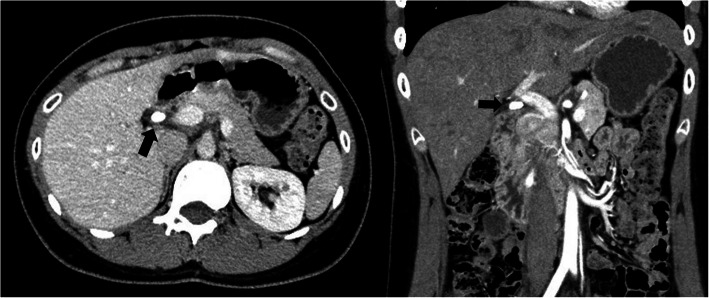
CT showing a cystic duct remnant calculus (arrow)
